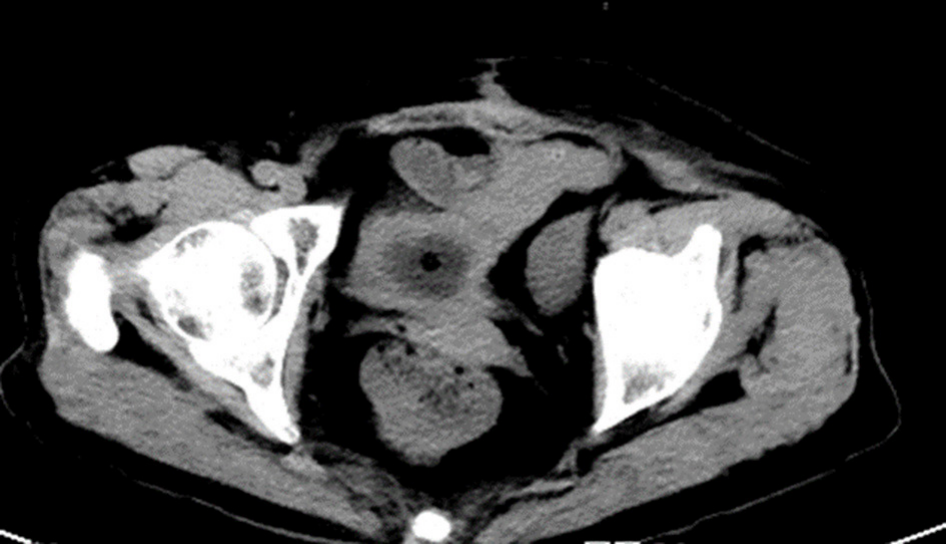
Coronal section CT scan of case 1. The CT scan demonstrated an indwelling catheter in the bladder and a small amount of fluid collection in the abdominopelvic cavity.
